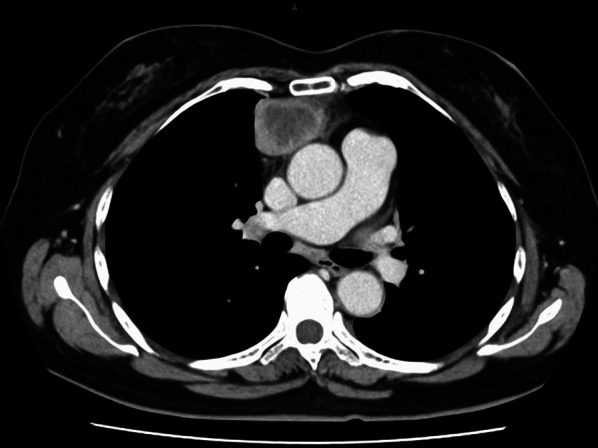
Chest computed tomography scan revealing a 55 × 43-mm exophytic heterogeneously enhancing mass exhibiting some areas of necrosis
